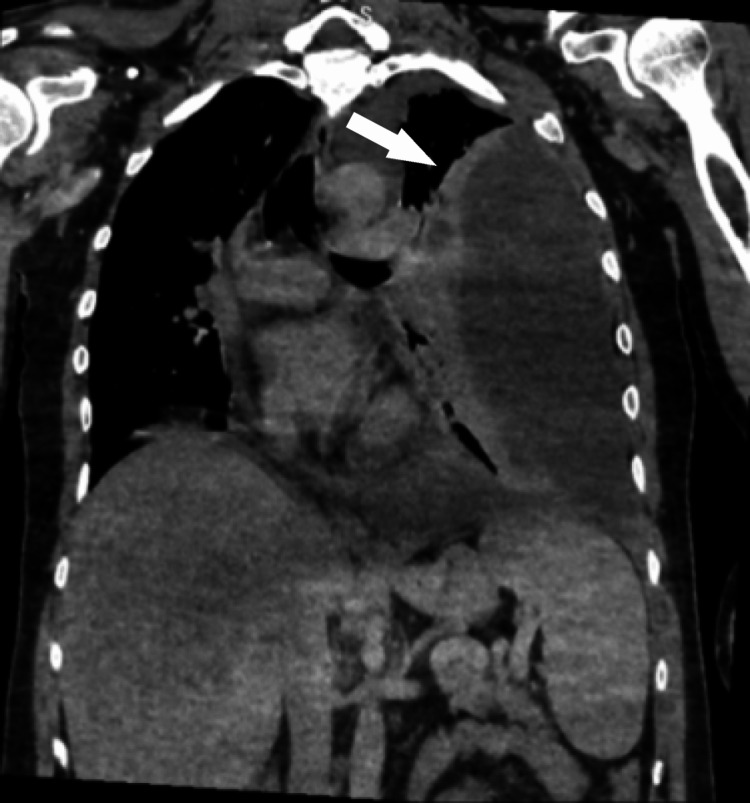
Coronal CT scan of the chest. Coronal CT image of the chest reveals a significant loculated left pleural effusion with mild, smooth pleural thickening and enhancement.
